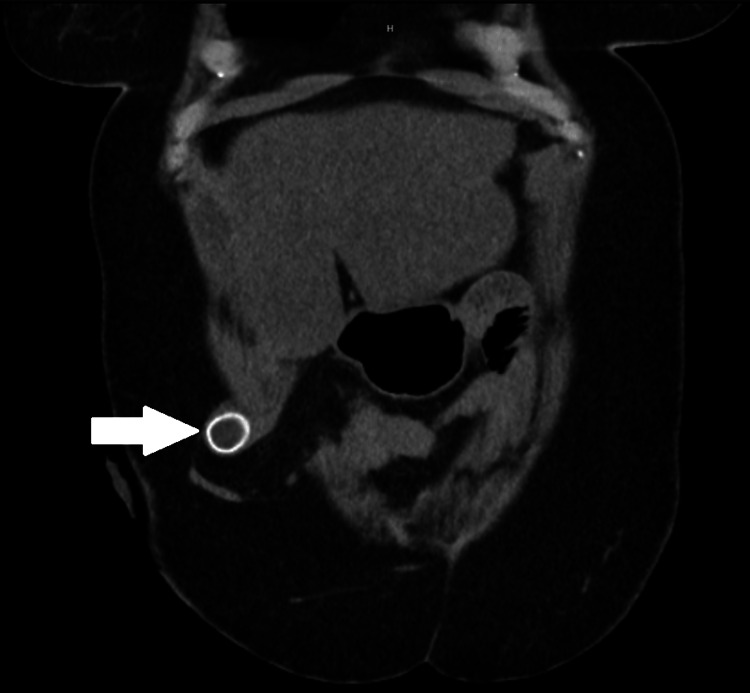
CT Abdomen and Pelvis with coronal view of parastomal gallbladder herniation White arrow demonstrating gallbladder with 2-cm gallstone herniating through parastomal hernia
